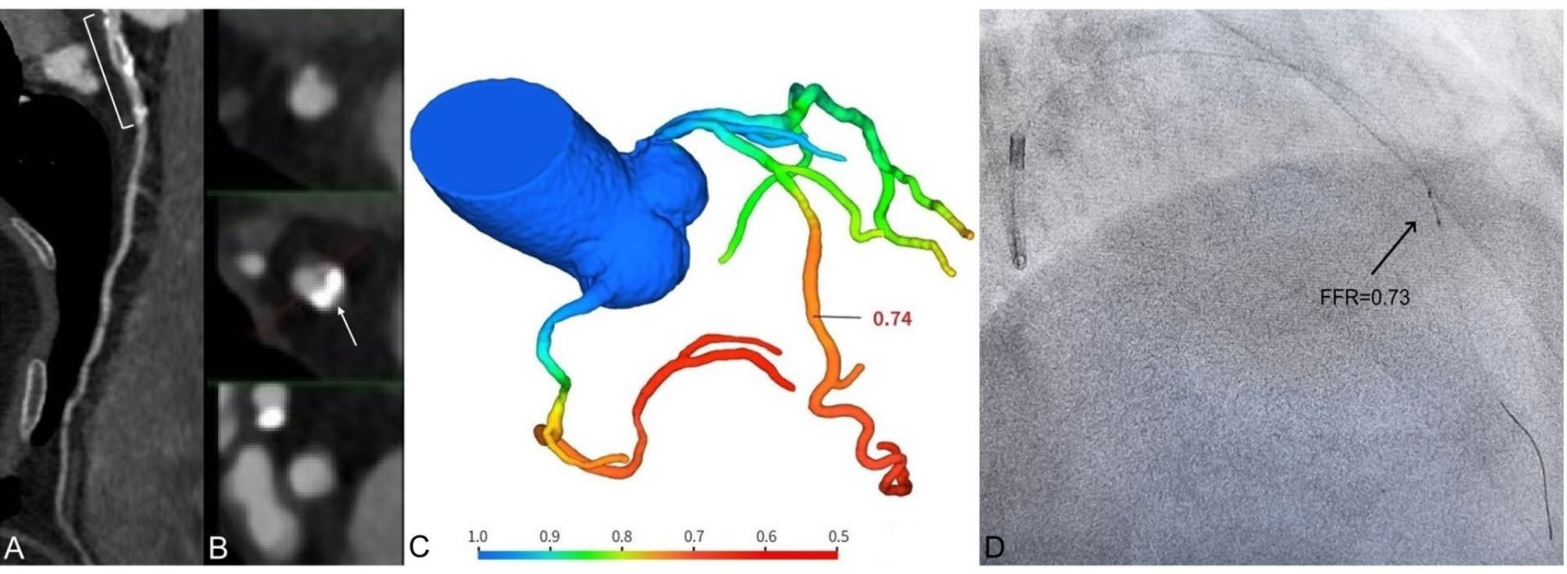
A 72-year-old patient underwent CTA, CT-FFR, and FFR examinations. (A) The axial and curved planar reformatted images of the calcified segment for LAD (the bracket); (B) The cross-sectional and curved planar reformatted images of the calcified segment for LAD (the arrow); (C) The CT-FFR value of the mid-segment of the LAD was 0.74; (D) The FFR value of the mid-segment of the LAD was 0.73. CTA, coronary computed tomography angiography; CT-FFR, computed tomography-derived computed fractional flow reserve; LAD, left anterior descending artery
